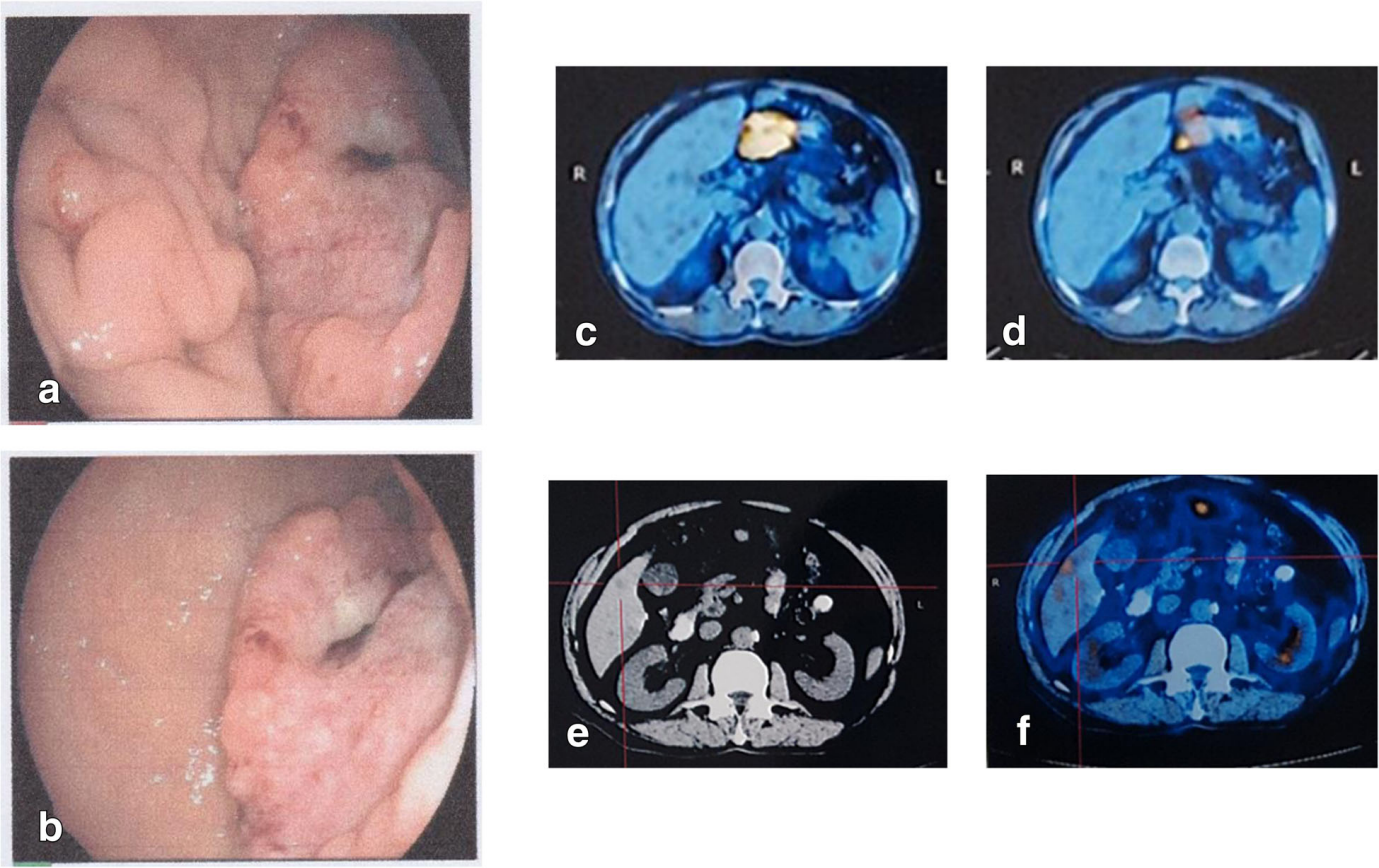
Endoscopy and PET/CT revealed GCLM. a. posterior wall of the antrum: worm-like erosion edges, visible bleeding from blood vessels and blood clots. b. small curvature of the antrum, white protrusions. c The lesion was located in the gastric antrum(SUVmax13.3), indicating GC. d Lymph node metastasis was observed below the gastric antrum (SUVmax3.3). d. Computed Tomography Image revealed a single liver metastasis, located in segments 6. e PET/CT identified localized radionuclide concentration (SUVmax3.5)
